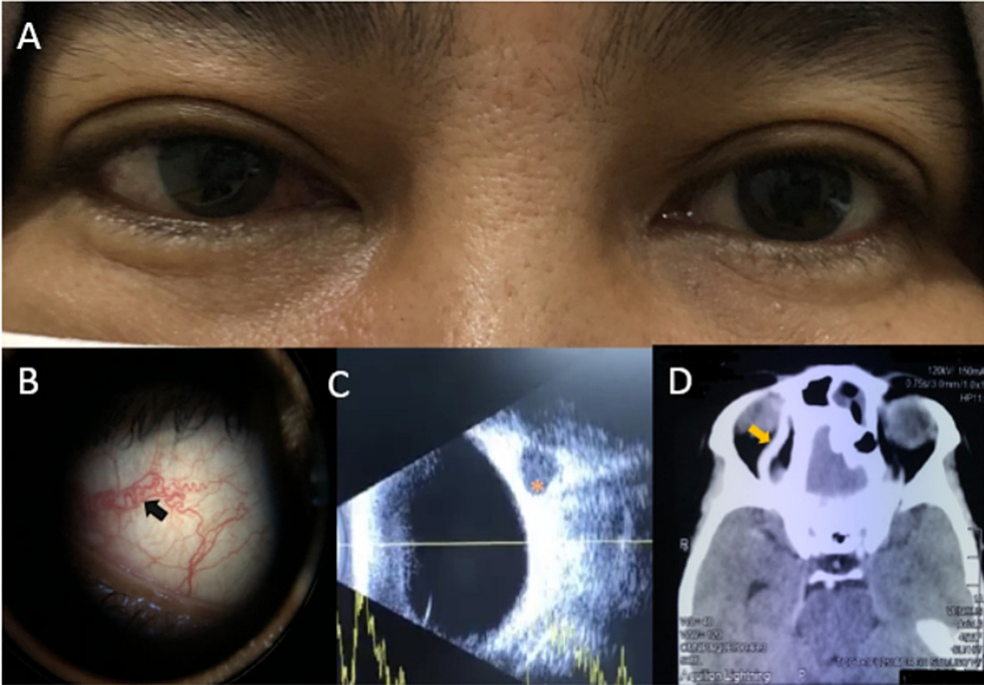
Ocular and radiological findings in Case 3. (A) Right eye redness with lid swelling. (B) Corkscrew vessels (black arrow) on slit-lamp examination. (C) Dilated right SOV (asterisk) in ultrasonography. (D) Dilated SOV (yellow arrow) in CTA.
